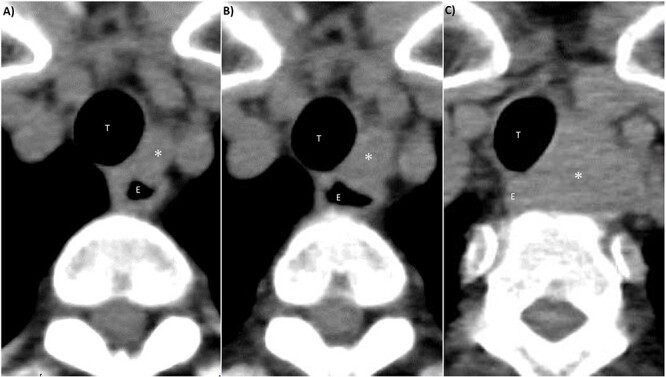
CT findings of left III lymph node metastasis. (A) 4 years after initial surgery, size: 9 mm × 7 mm. (B) 5 years after initial surgery, size: 14 mm × 10 mm. (C) 7 years after initial surgery, size: 32 mm × 27 mm. ^*^: left III lymph node, T: trachea, E: esophagus.
